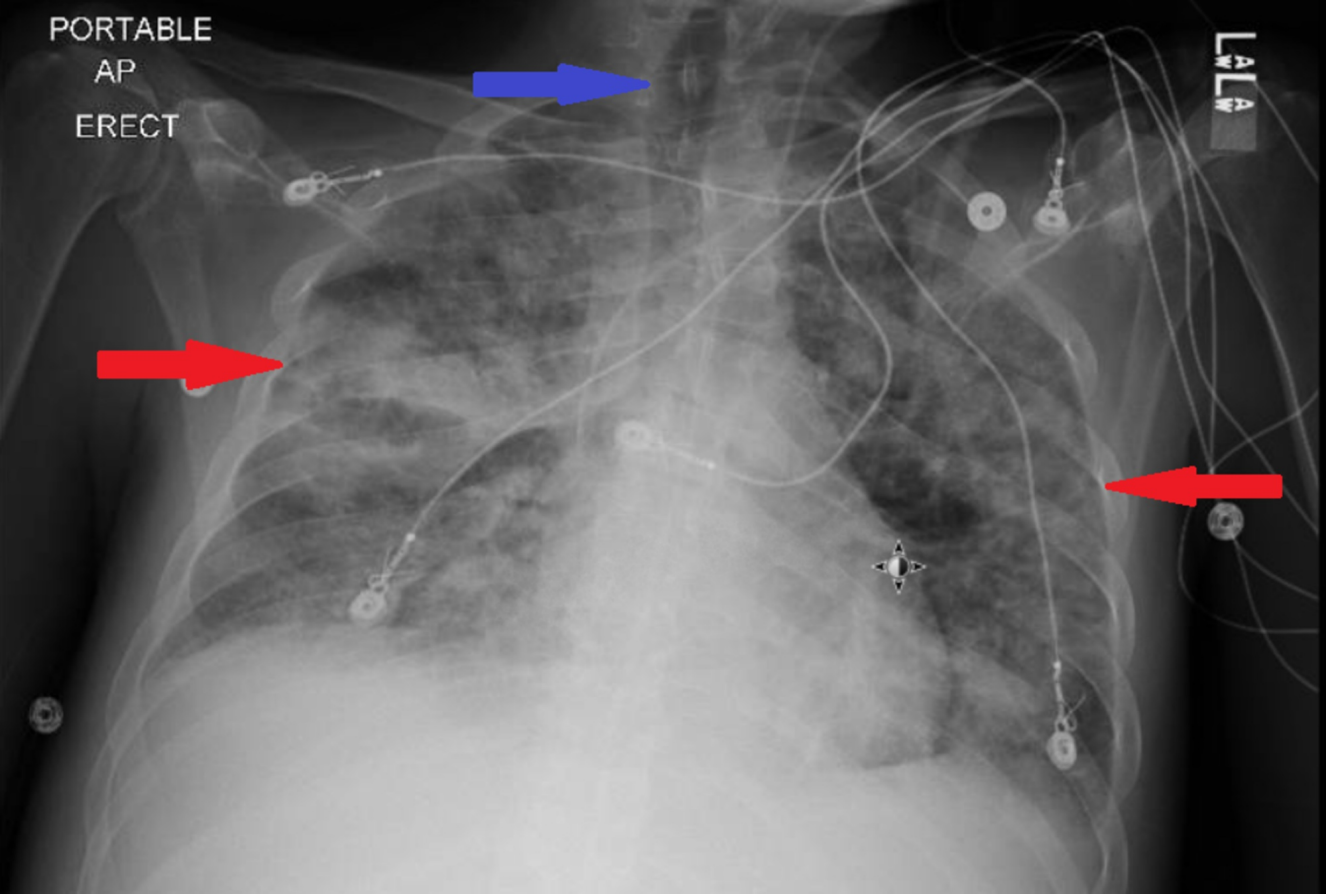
Chest x ray on day 5 demonstrating a bilateral infiltrate (red arrows), radiographic changes can lag behind clinical improvement. Endotrachial tube is no longer visible (blue arrow) .
